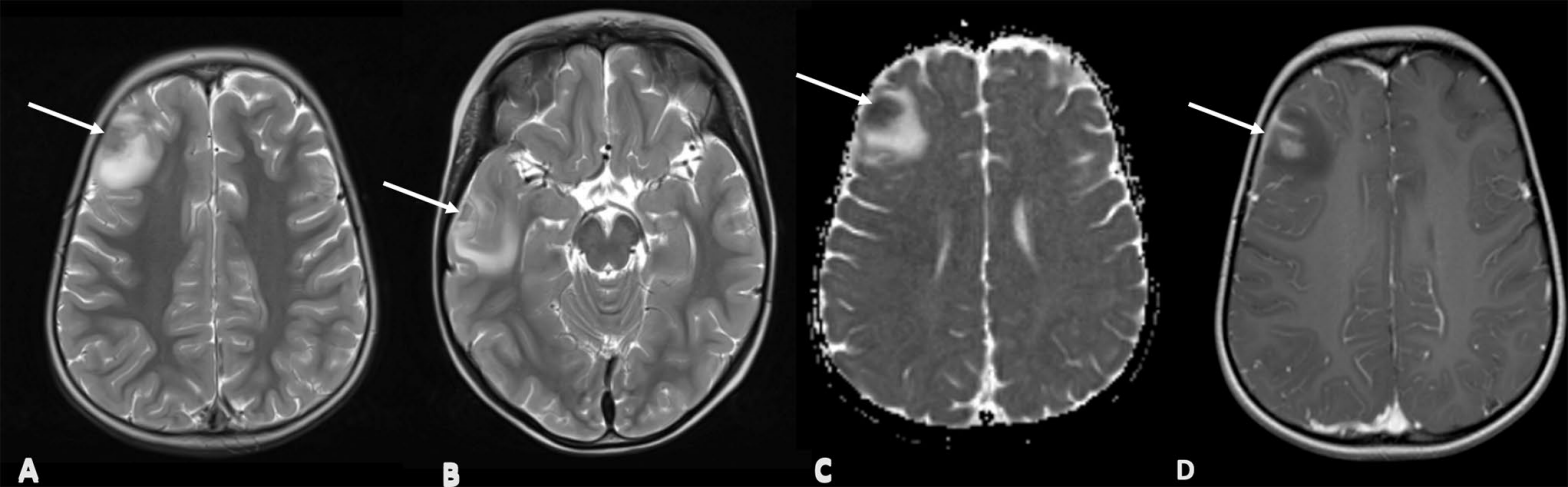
MRI brain images of P5 showing lesions in the right frontal and right temporal lobes with peripheral enhancement and surrounding oedema. Axial T2 weighted images (A, B), axial ADC diffusion map (C) and axial post-contrast T1 weighted images (D) show two lesions (arrows) in the right frontal and temporal lobes (arrows) with T2 hypointensity with diffusion restriction and enhancement in keeping with high cellularity expected in lymphoproliferative disorder. MRI – magnetic resonance imaging, ADC – apparent diffusion coefficient
